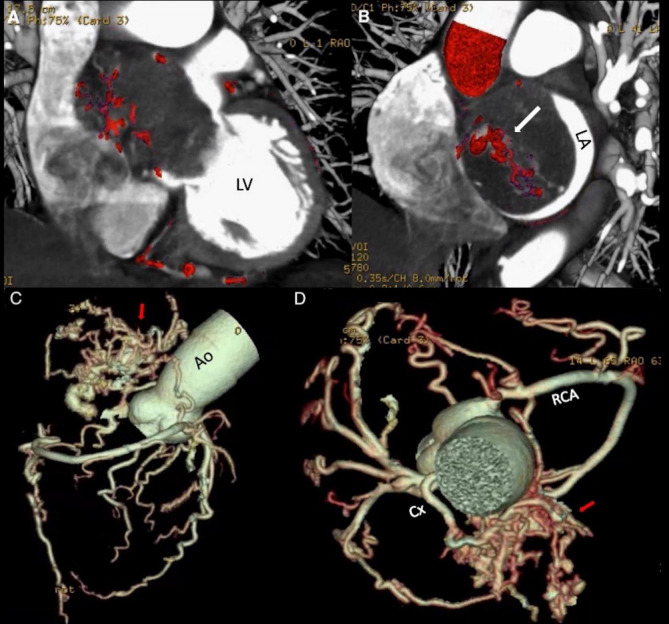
(A,B) Coronary Computed Tomography Angiography. Hypodense mass located in the left atrium attached to the interatrial septum, with a dense network of blood vessels within. The intra- atrial mass protrudes through the mitral valve into the left ventricle during diastole, causing impairment of the atrial emptying and mitral valve closure. (C,D) Coronary Computed Tomography Angiography reconstruction. Complex blood vessel network (arrows) anastomosed to branches of the circumflex artery and the right coronary artery (Supplementary Video 3). Ao, aorta; Cx, circumflex artery; LA, left atrium; LV, left ventricle; RCA, right coronary artery.
